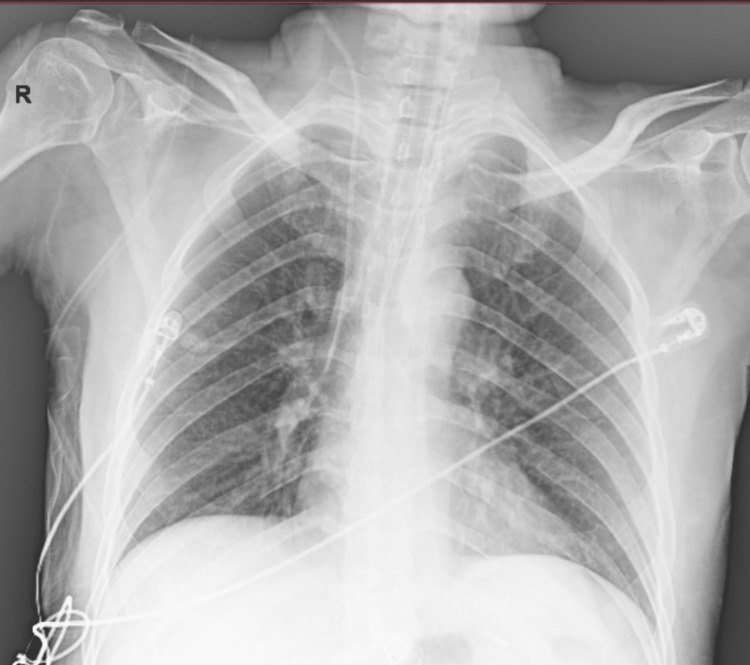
Bedside chest radiograph on Day 5 The image shows reduction in bilateral air-space opacities with improved lung aeration, suggestive of resolving pneumonia
